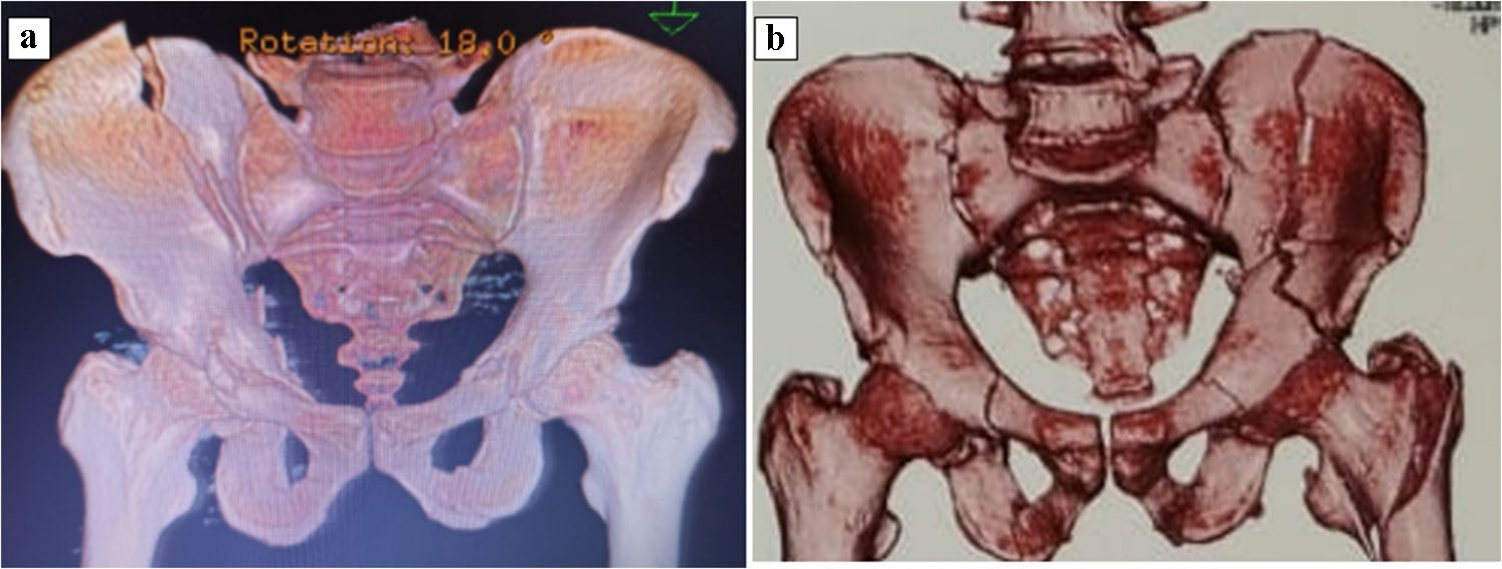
shows preoperative CT 3d that show: a-associated BC, and b- anterior column posterior hemi-transverse fracture acetabulum, respectively
